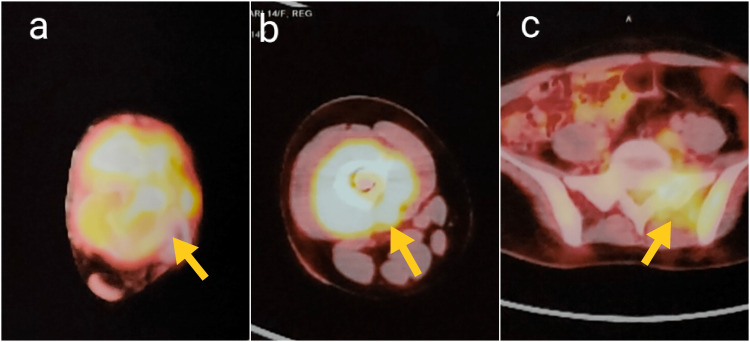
PET/CT images (a, b, c) demonstrate increased tracer uptake in the left talus, right shaft of the femur, and left sacrum and hemi pelvis (yellow arrows). PET/CT: Positron emission tomography/computed tomography
